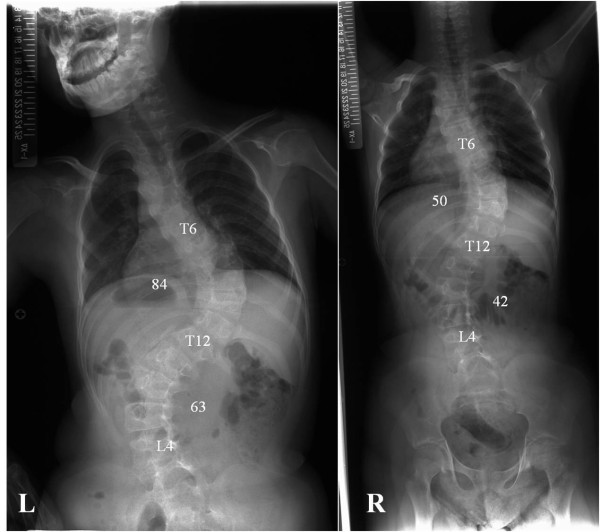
Preoperative full-length spine radiographs. Left: standing, Right: traction.
